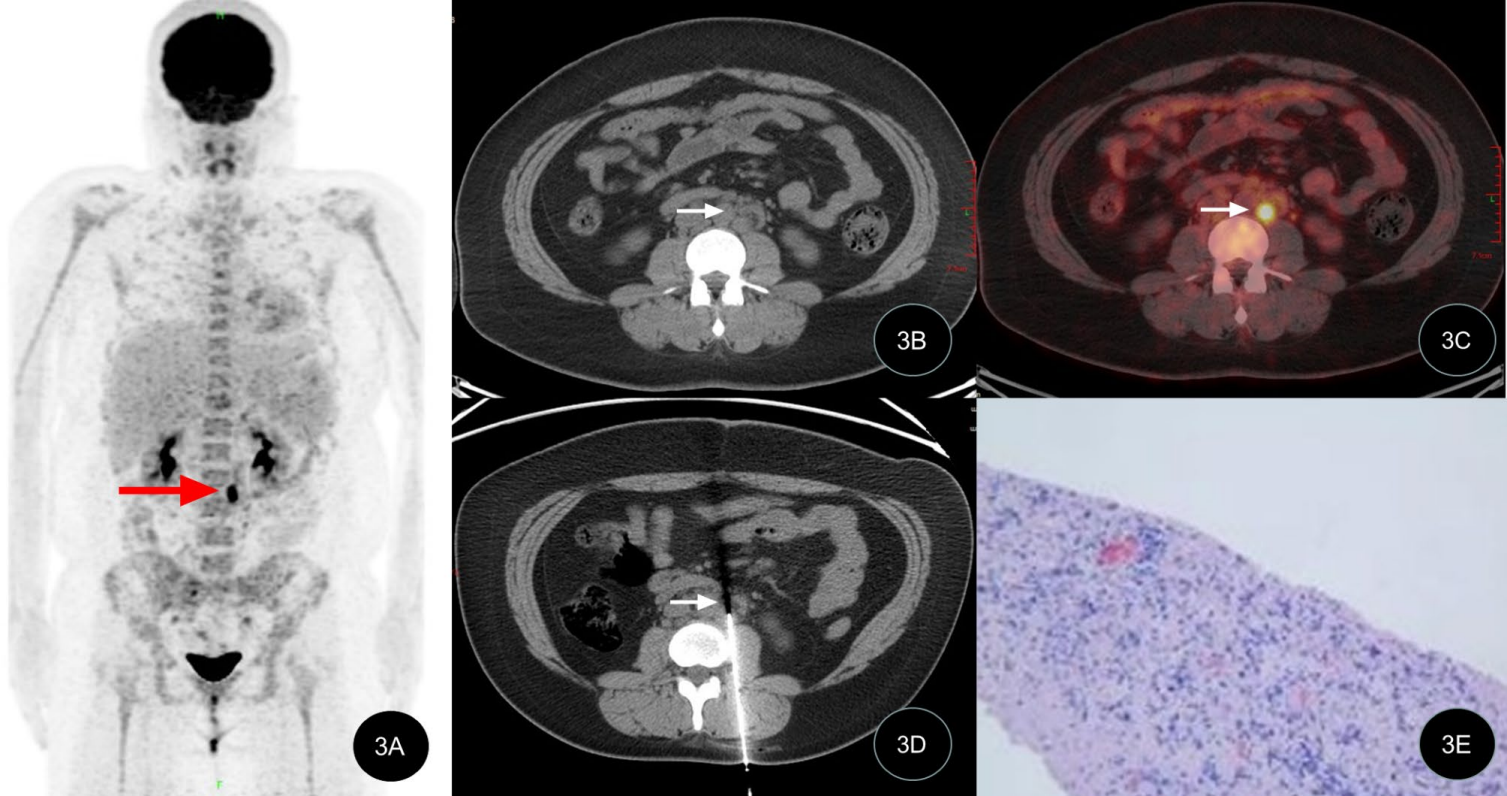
PET/CT-guided targeted retroperitoneal masses biopsy Lymphoproliferative disorders; The patient (22-year-old female) was admitted due to mild fever with abdominal pain. The PET/CT MIP image, PET/CT fusion CT image, puncture biopsy image, and biopsy pathological image are shown. 3A MIP image shows abnormal metabolism increase at the left side of the lumbar spine (indicated by red arrow); 3B, 3C PET/CT fusion CT image shows increased metabolism at the retroperitoneal lymph node; 3D Puncture biopsy of the target area of the retroperitoneal lymph node under PET/CT guidance; 3E Pathological examination shows kikuchi disease (HE × 100)
